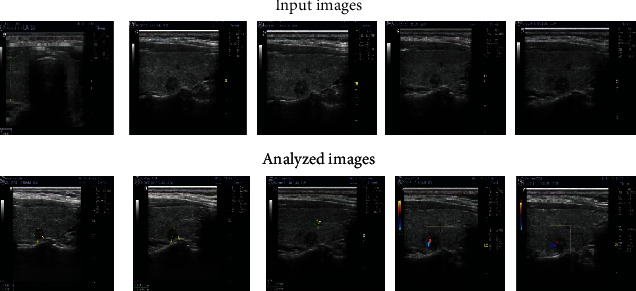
Samples of input images and analyzed images observed during ultrasound diagnosis of thyroid nodules.
